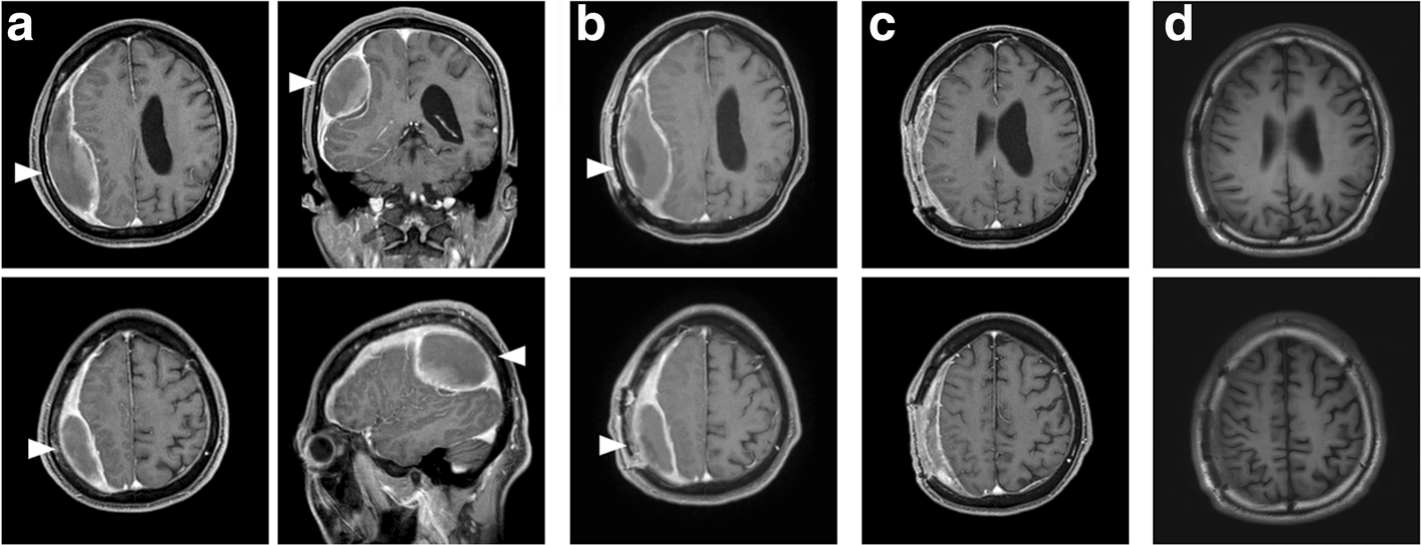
Nuclear magnetic resonance imaging of the head and brain. a Gadolinium-enhanced T1-weighted imaging (Gd T1WI) at the time of admission showed right subdural empyema (*white arrowhead*, 106 × 33 × 53 mm) with mixed low intensity. b Gd T1WI after 3 weeks of treatment. The size of the abscess was not reduced (*white arrowhead*, 109 × 35 × 60 mm). c Gadolinium-enhanced T1-weighted imaging 2 weeks after the second drainage and antimicrobial drug change to meropenem. The abscess was decreased in size. d T1-weighted imaging without contrast, 1 year after the treatment. There was no recurrence of the abscess
